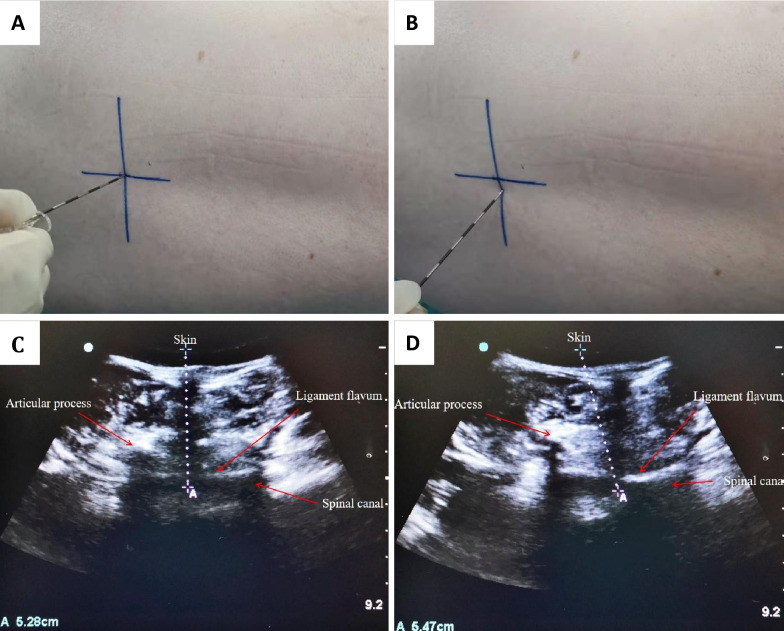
A Location of median approach on the skin. B Location of paramedian approach on the skin. C The distance from the skin to the epidural space guided by ultrasound in the median approach group. D The distance from the skin to the epidural space guided by ultrasound in the paramedian approach group
